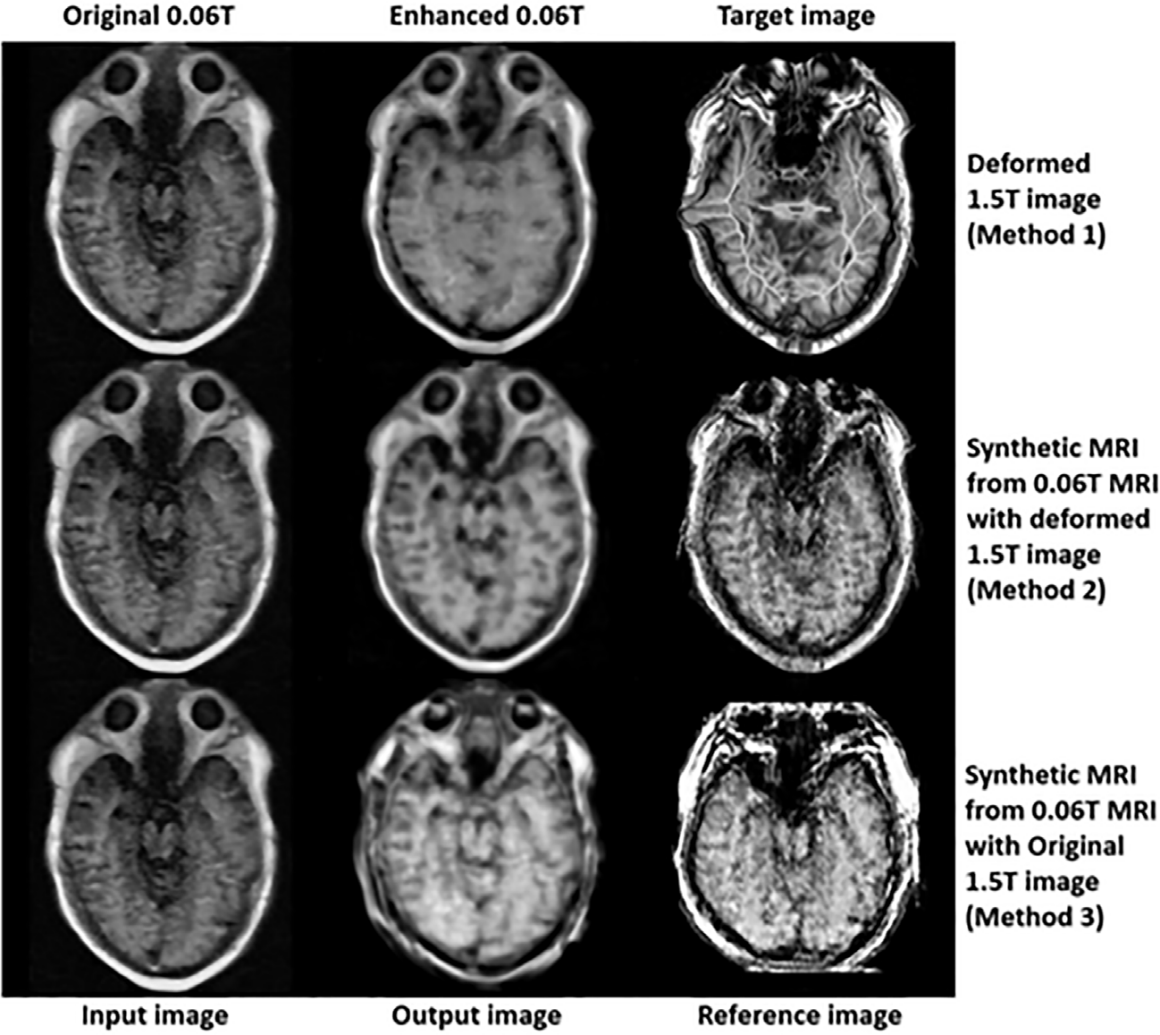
Results of enhanced MRI of slice no. 10 in step 3 (conventional-GAN model).
